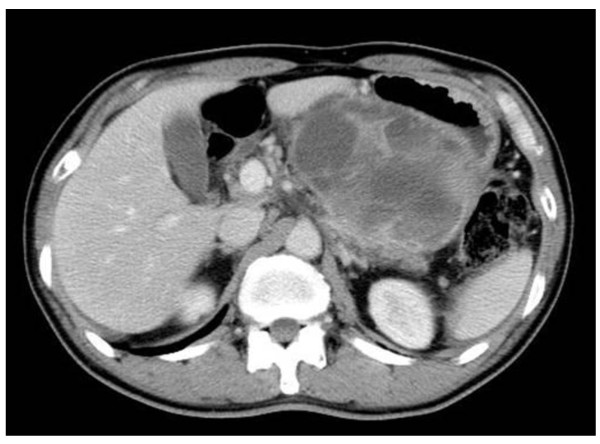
Abdominal CT showing the large cystic tumor in the upper left quadrant of the abdomen. A computed tomography scan of the abdomen revealed a large cystic mass appeared to originate from the pancreatic tail.
